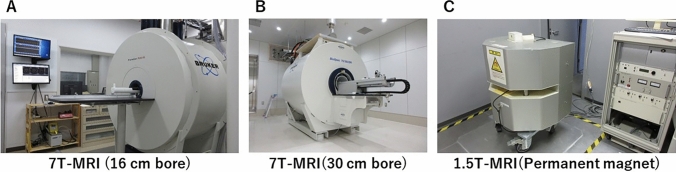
A Preclinical 7 T MRI (16 cm small bore, helium autonomy 150 days with nitrogen compressor system). B Preclinical 7 T MRI (30 cm wide bore, zero helium boil-off technology, nitrogen-free auto-bed system). C Preclinical 1.5 T MRI (open permanent magnet gradient, with the motor-driven automatic positioning system AutoPac™)
